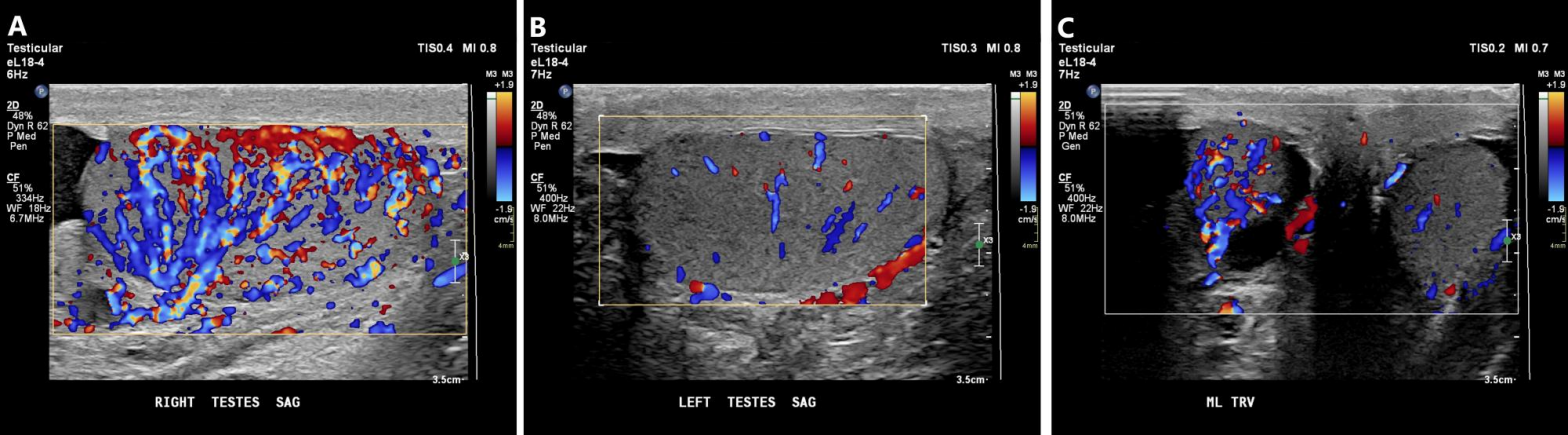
Orchitis of the right testis. (A) The right testis is enlarged and demonstrates hyperemia on Doppler interrogation. (B) The contralateral left testis has a normal appearance and blood flow. (C) When both testes are viewed simultaneously, the right testicular hyperemia is more easily appreciated.
